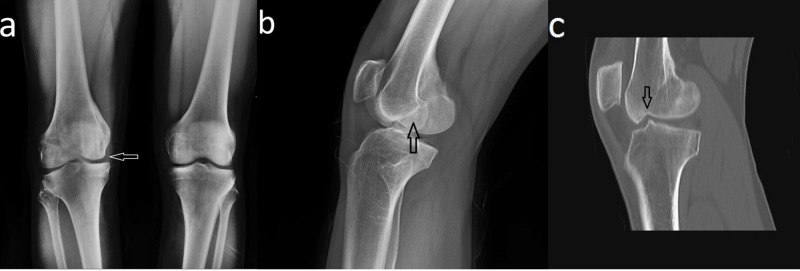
Pre-operative radiographic images of the patient (a,b) Anteroposterior and lateral radiographic images of the patient show a coronal plane fracture (type III) of the medial femoral condyle of the right knee (arrow). (c) Computed tomography image shows the incongruity of the articular surface (arrow).
